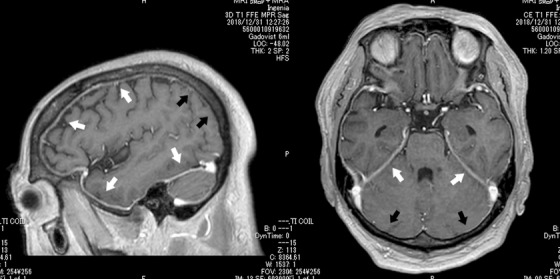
Cranial MRI (T1 fast field echo method, enhanced with gadolinium)
